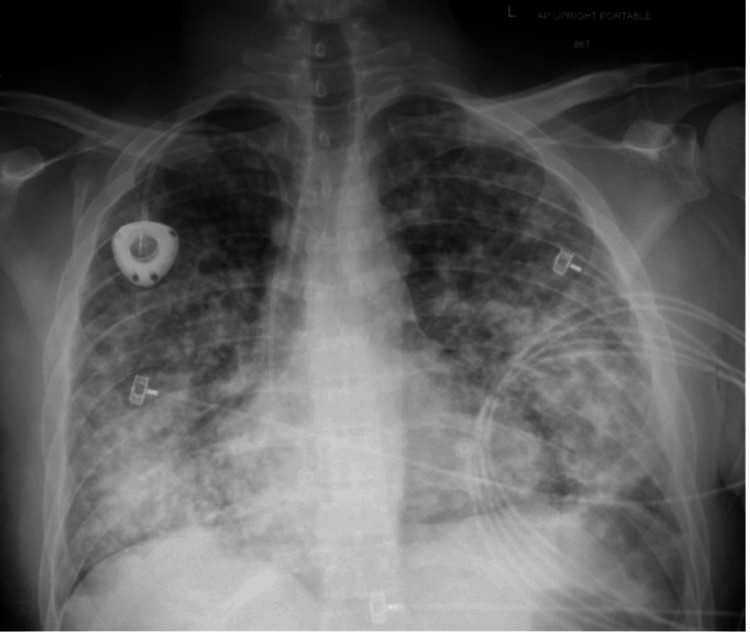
Worsening pulmonary Kaposi sarcoma (KS) on chest X-ray Chest X-ray showing worsening pulmonary nodules indicative of pulmonary KS progression.
